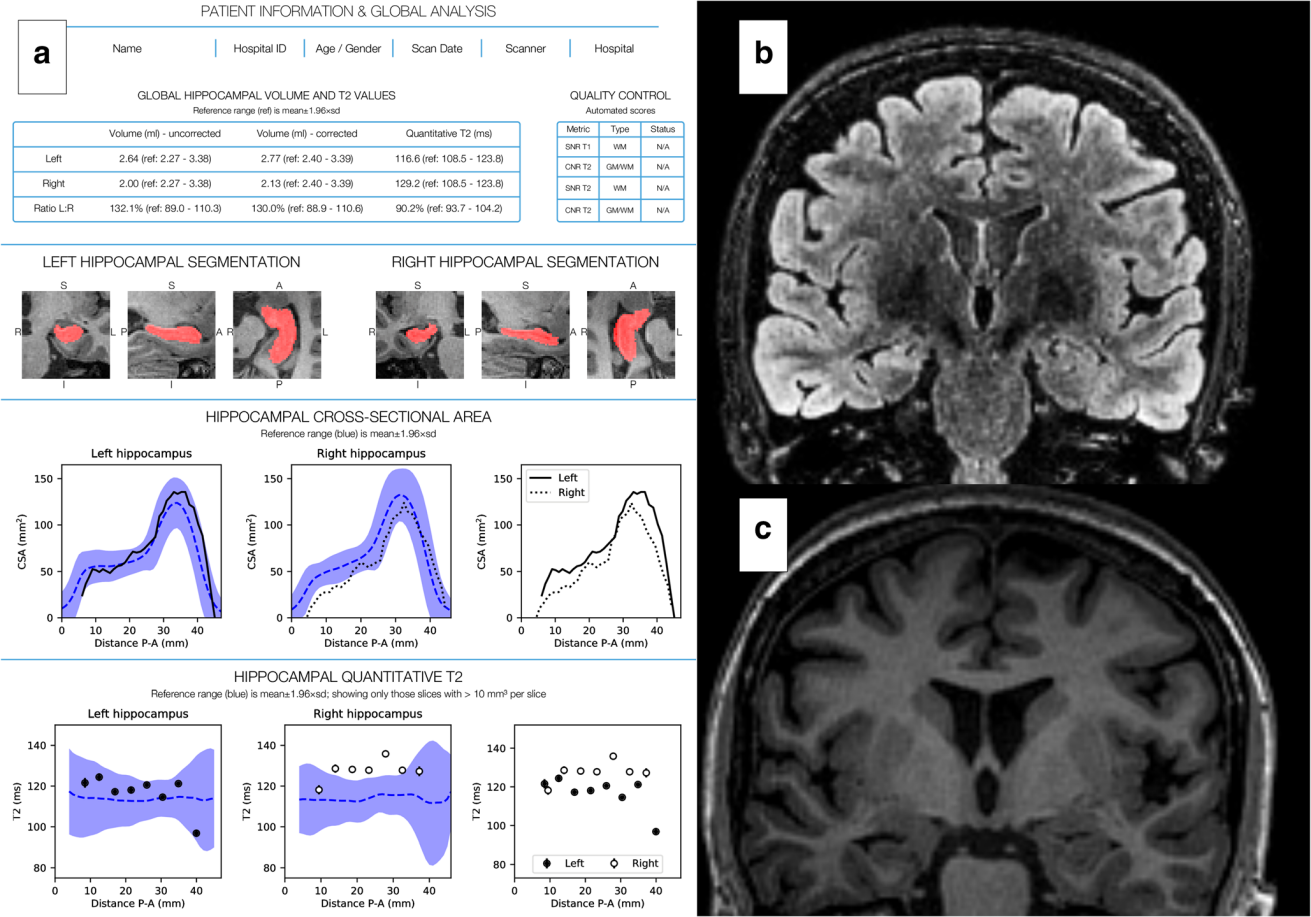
QReport and MR images of a patient with right HS. a QReport displaying patient information; global analysis including global measurements and left:right ratios with normative reference ranges in brackets; quality control; snapshots of hippocampal segmentations; graphs for hippocampal cross-sectional area and qT2 posterior-anterior (P-A) along the hippocampal long axis. Graphical display: black lines or dots represent patient’s values, blue dotted line and blue band represent normative data mean ± 1.96SD, graphs with no reference data are a representation of the patient’s left:right ratio. b Coronal FLAIR image showing right hippocampal hyperintensity. c Coronal T1-weighted image showing right hippocampal volume loss
